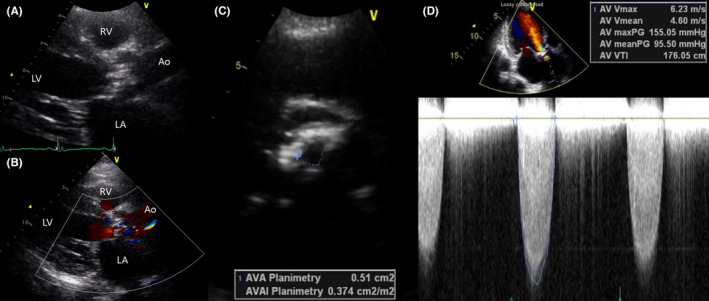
Initial transthoracic echocardiography (TTE) findings. (A, B) TTE showed left ventricular hypertrophy and degenerative aortic valve with severe calcification. (C) The aortic valve area (AVA) was 0.51 cm^2^ on the planimetry. (D) The mean systolic pressure gradient of aortic valve was 95.50 mmHg, and peak aortic jet velocity was 6.23 m/s on the continuous wave Doppler.
